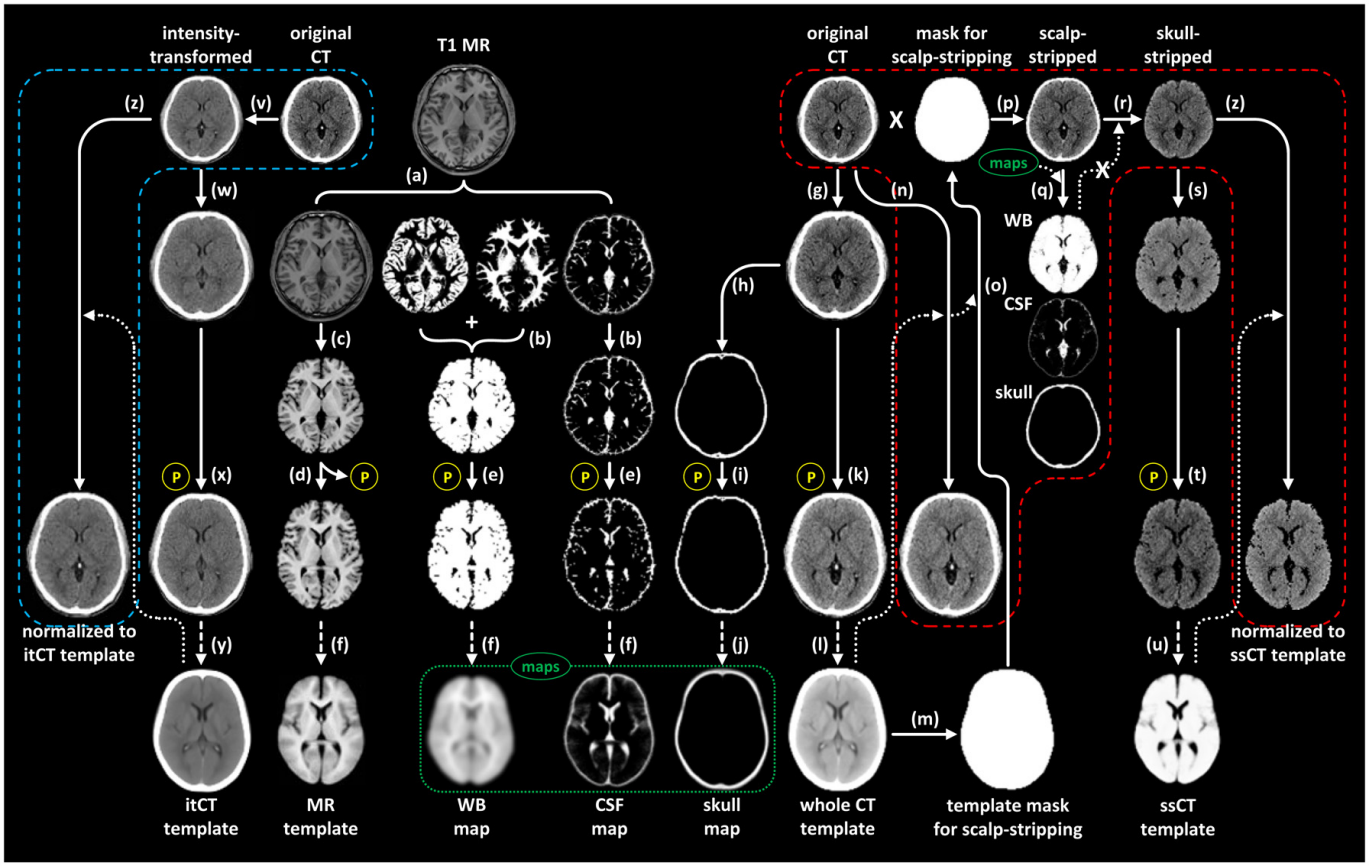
Image processing steps for acquiring skull-stripped CT (ssCT) and intensity-transformed CT (itCT) templates. (a) Inhomogeneity correction and segmentation of MR, (b) creation of whole brain and CSF masks, (c) skull-stripping of inhomogeneity-corrected MR, (d) spatial normalization of skull-stripped MR to MNI template for skull-stripped MR, (e) spatial normalization of masks by applying normalization parameter, (f) creation of probabilistic maps for whole brain and CSF by averaging tissue masks, (g) coregistration of CT to inhomogeneity-corrected MR, (h) creation of skull mask, (i) spatial normalization of skull mask, (j) creation of probabilistic map for skull, (k) spatial normalization of coregistered CT, (l) creation of whole CT template by averaging, (m) creation of template mask for scalp-stripping, (n) spatial normalization of CT to whole CT template, (o) inverse normalization of template mask for scalp-stripping to create individual mask for scalp-stripping, (p) creation of scalp-stripped CT by applying mask, (q) segmentation of scalp-stripped CT into three tissue type by using probabilistic maps, (r) creation of ssCT by applying mask for whole brain segment, (s) coregistration of ssCT to MR by applying parameter coregistering original CT to MR, (t) spatial normalization of coregistered ssCT, (u) creation of ssCT template by averaging, (v) intensity transformation of original CT, (w) coregistration of itCT to MR, (x) spatial normalization of coregistered itCT, (y) creation of itCT template by averaging, and (z) spatial normalization of individual ssCT and itCT to specific CT templates. The processing steps inside the red (ssCT) and blue (itCT) dashed lines represent the image processing steps required for the spatial normalization of CT images with created CT templates.
